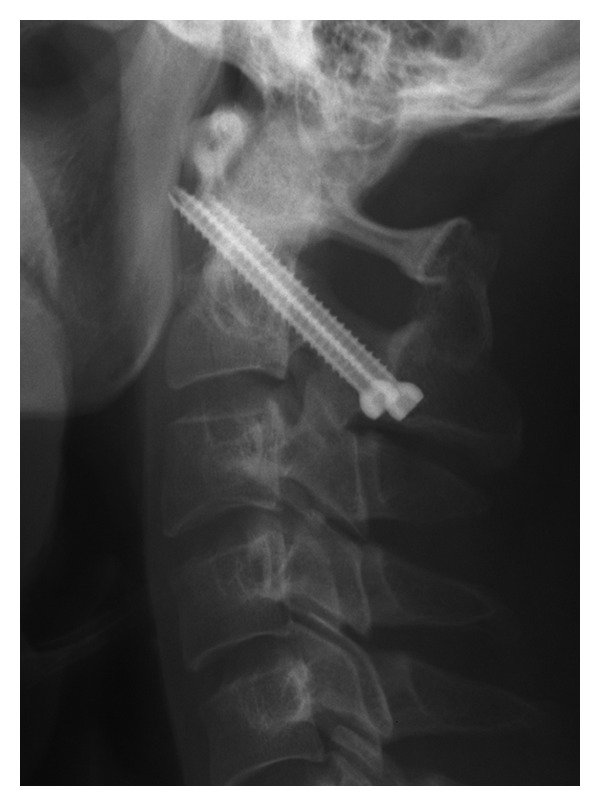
Postoperative cervical spine plain X-ray images. Atlantoaxial subluxation was improved with the adhesion of grafted bone.
